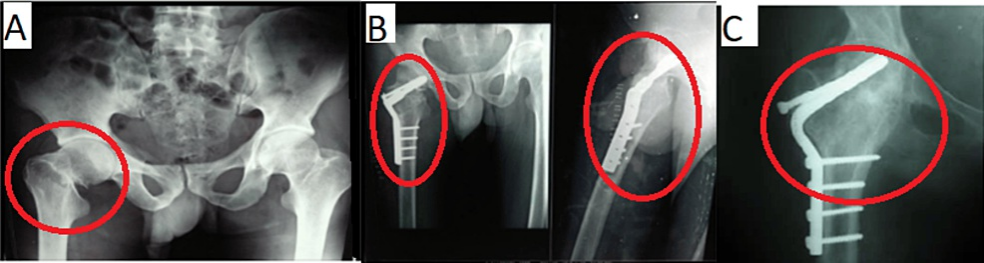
Pre- and post-operative radiographs with radiographic union (A) Pre-operative. (B) Six-month follow-up. (C) Twenty-month follow-up
